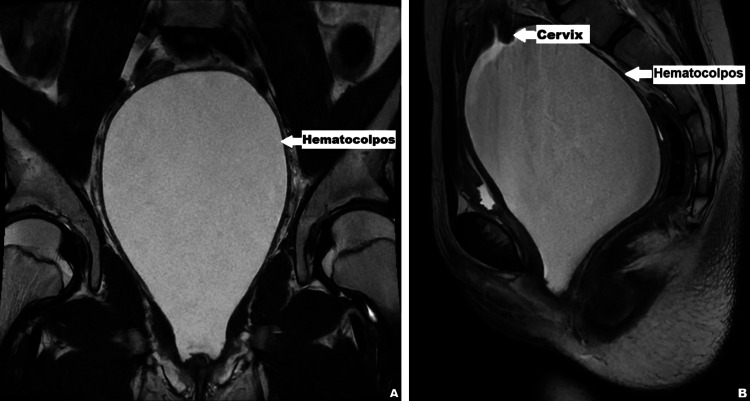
Coronal (A) and sagittal (B) MRI images demonstrating a distended vaginal cavity consistent with hematocolpos. The hyperintense signal on T2-weighted imaging indicates blood collection within the vagina, secondary to outflow tract obstruction. MRI: magnetic resonance imaging.
